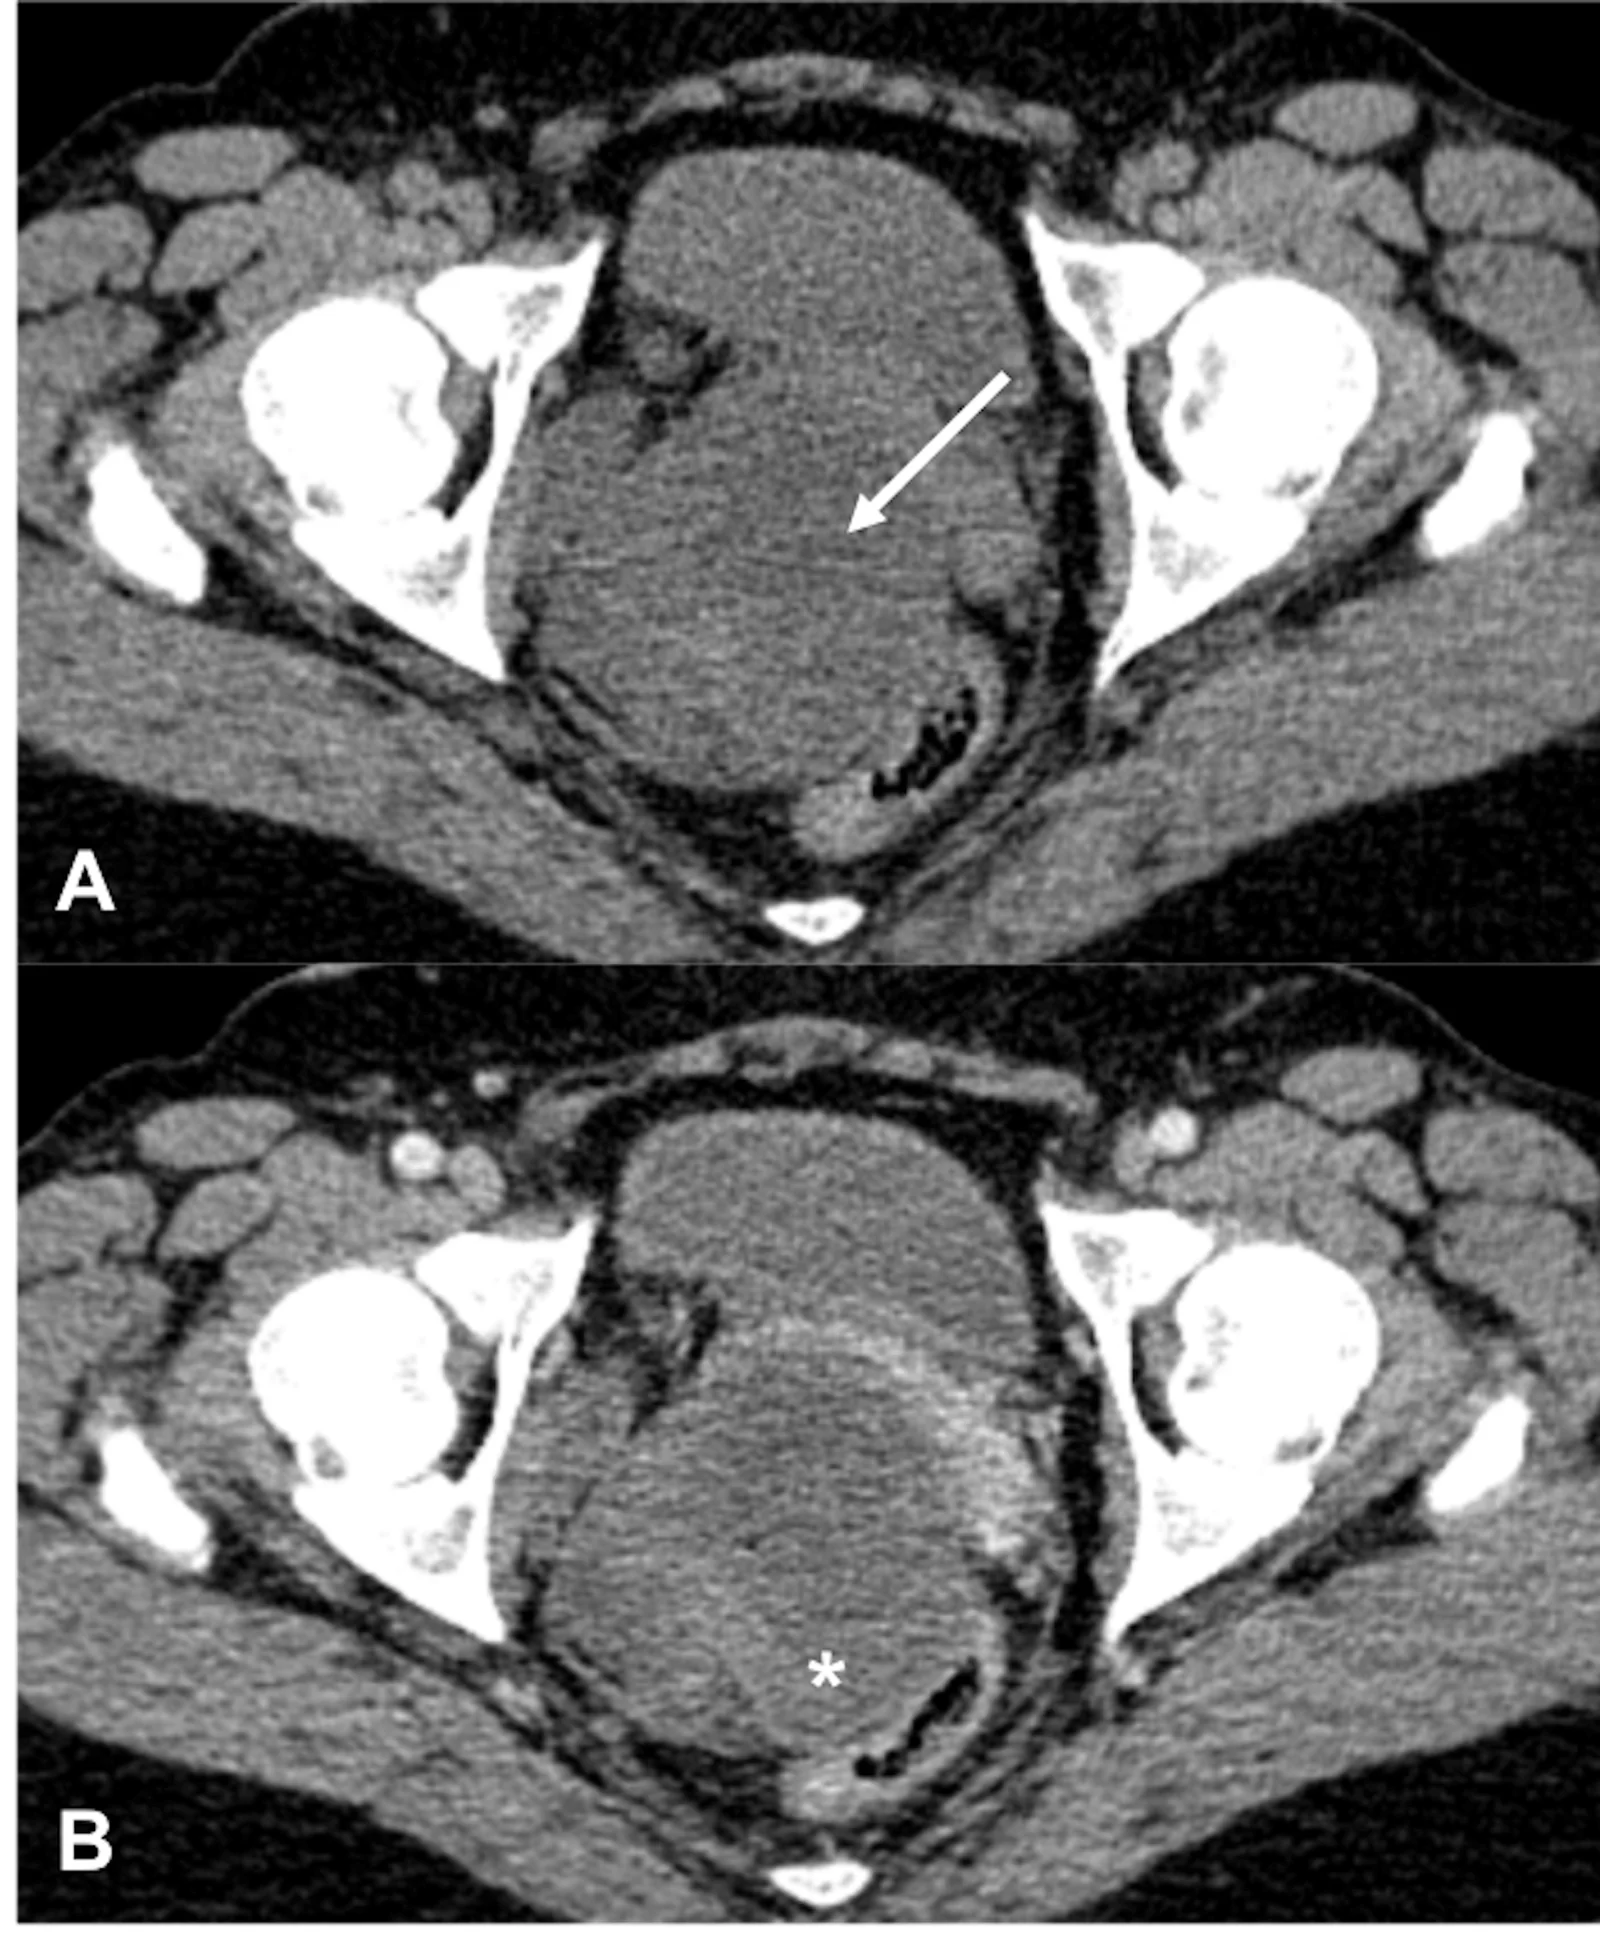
Axial CT images of the pelvic tumor A) Unenhanced CT demonstrates a well-circumscribed hypodense mass centered in the rectovaginal space (arrow), measuring 77 × 69 mm (transverse × anteroposterior). The mass displaces the vagina and urinary bladder anteriorly and the rectosigmoid junction posterolaterally to the left. B) Following intravenous iodinated contrast administration, the mass demonstrates mild heterogeneous enhancement (*).
